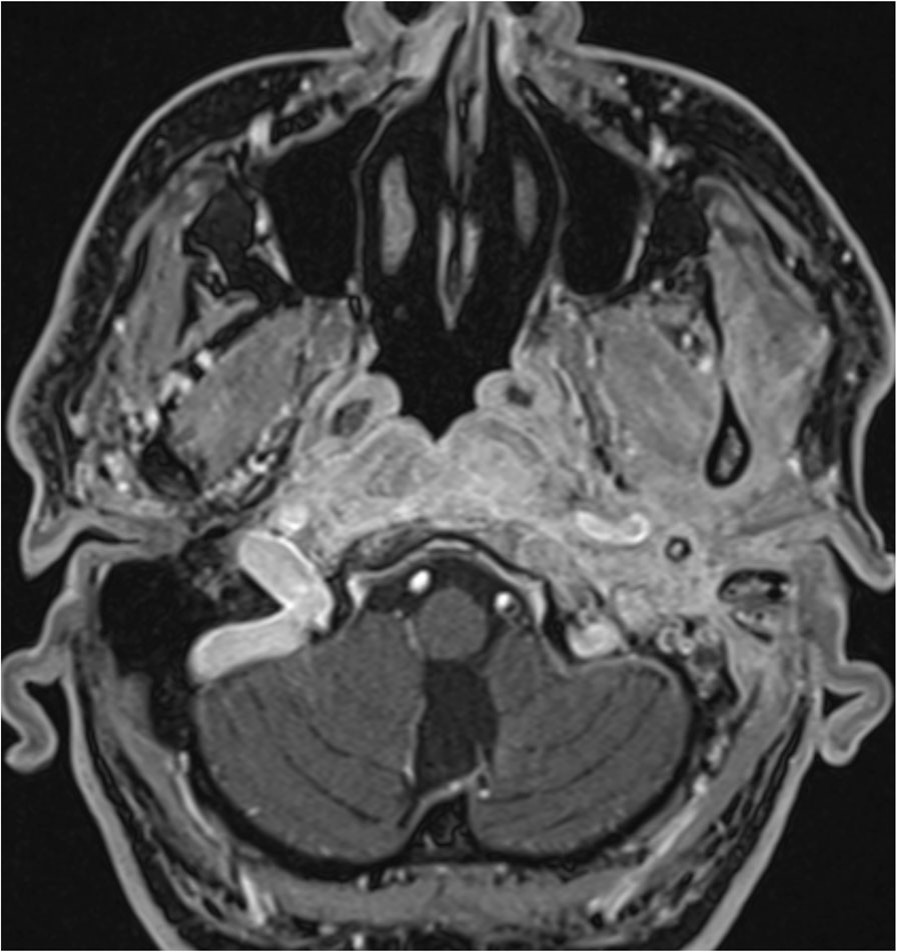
Day 93–177. Gadolinium-enhanced MRI, axial plane, T1-weighted, with fat suppression. The signal increases homogeneously on the left side in retrostyloid and pre-styloid regions, longus colli muscle, and Eustachian tubes on both sides. The signal mildly and homogeneously increases in the temporomandibular joint, lateral pterygoid, and masseter muscles on the left side. Bone marrow shows signs of infiltration in the basal part of the occipital bone
